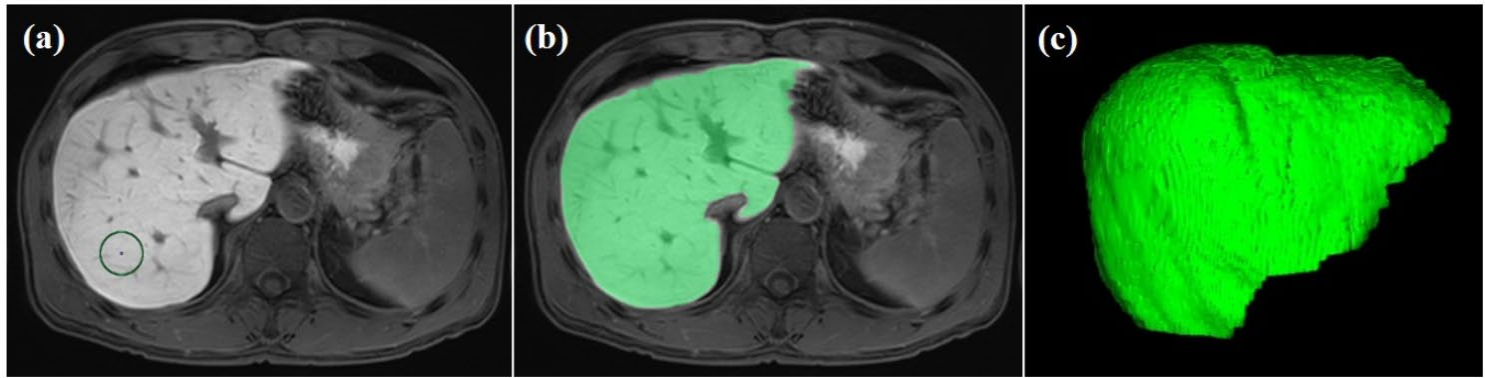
Image analysis using the three-dimensional volumetric system on magnetic resonance imaging with gadolinium-ethoxybenzyl-diethylenetriamine pentaacetic acid. (a) The investigator places a small volume of interest in liver parenchyma. (b) Liver parenchyma is semi-automatically extracted from the initial volume-of-interest seed. (c) The three-dimensional whole-liver volume is extracted, and the average whole-liver signal intensity is calculated automatically
